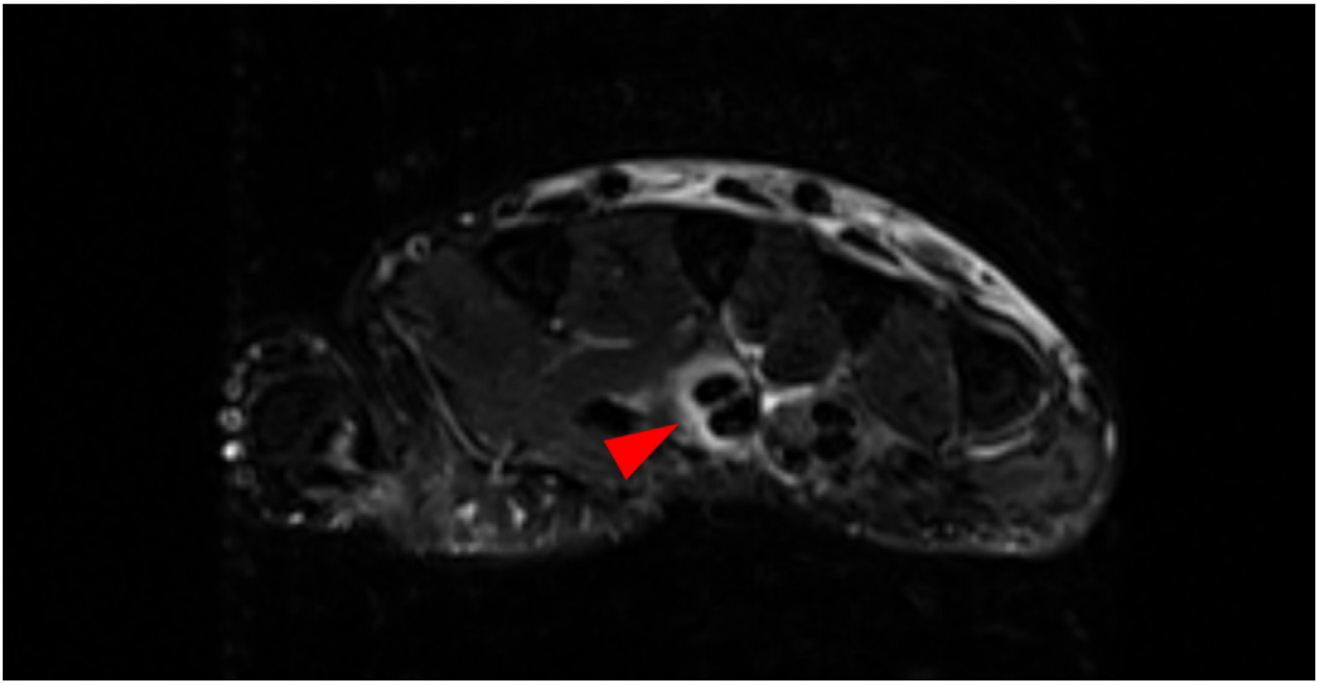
MRI on admission showed high-signal areas in the right middle flexor tendon and subcutaneous tissue of the right middle finger.
